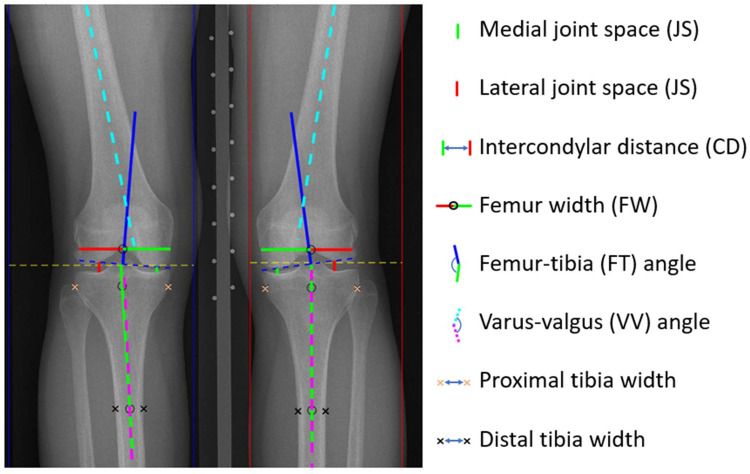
Dimensions and angles measured from the load-bearing anterior-posterior knee radiographs. The yellow horizontal dashed line was used for setting the approximated proximo-distal center of the tibiofemoral joint.
